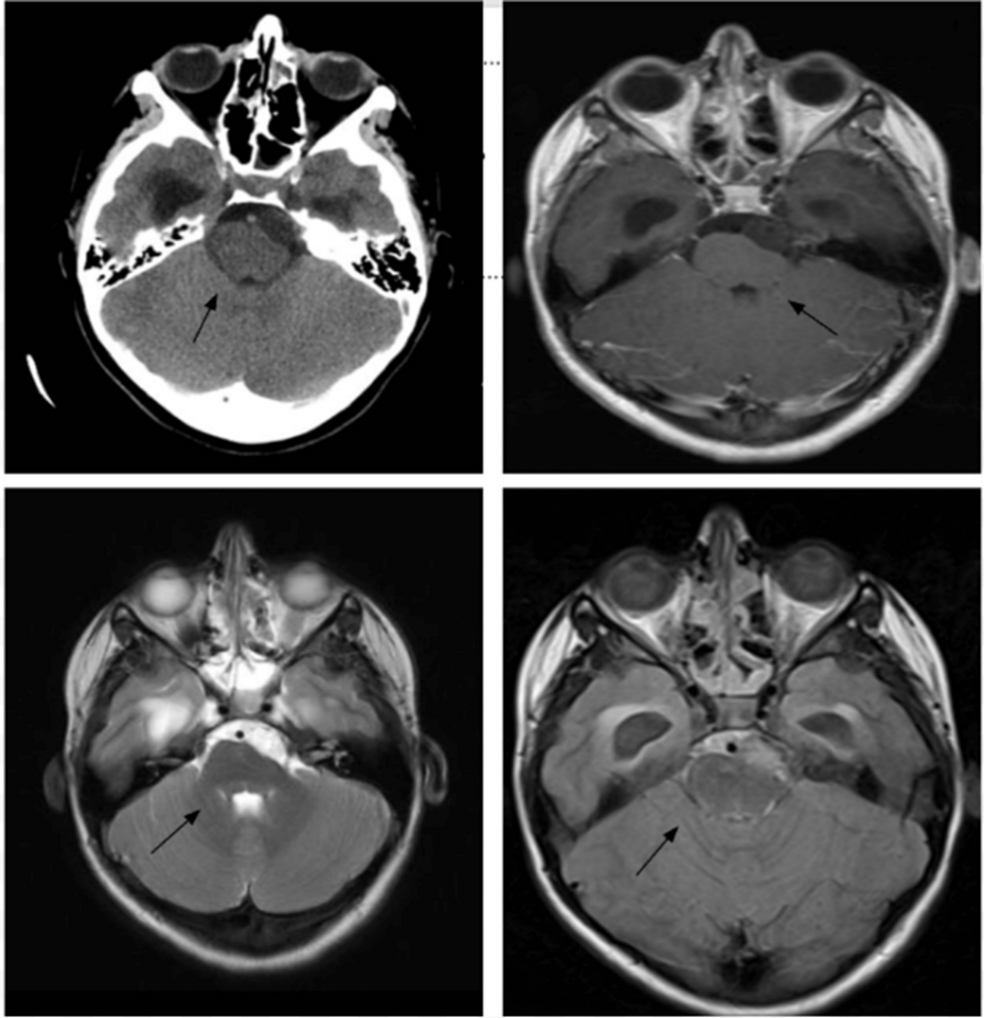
MRI axial T2-weighted images Axial CT scan showing a hypodense mass in the prepontine and cerebellopontine cistern plus hydrocephalus (superior left). This mass was hypointense in T1 (superior right), hyperintense in T2 (inferior left), and hyperintense in FLAIR (inferior right) suggesting an epidermoid tumor. FLAIR: fluid-attenuated inversion recovery
